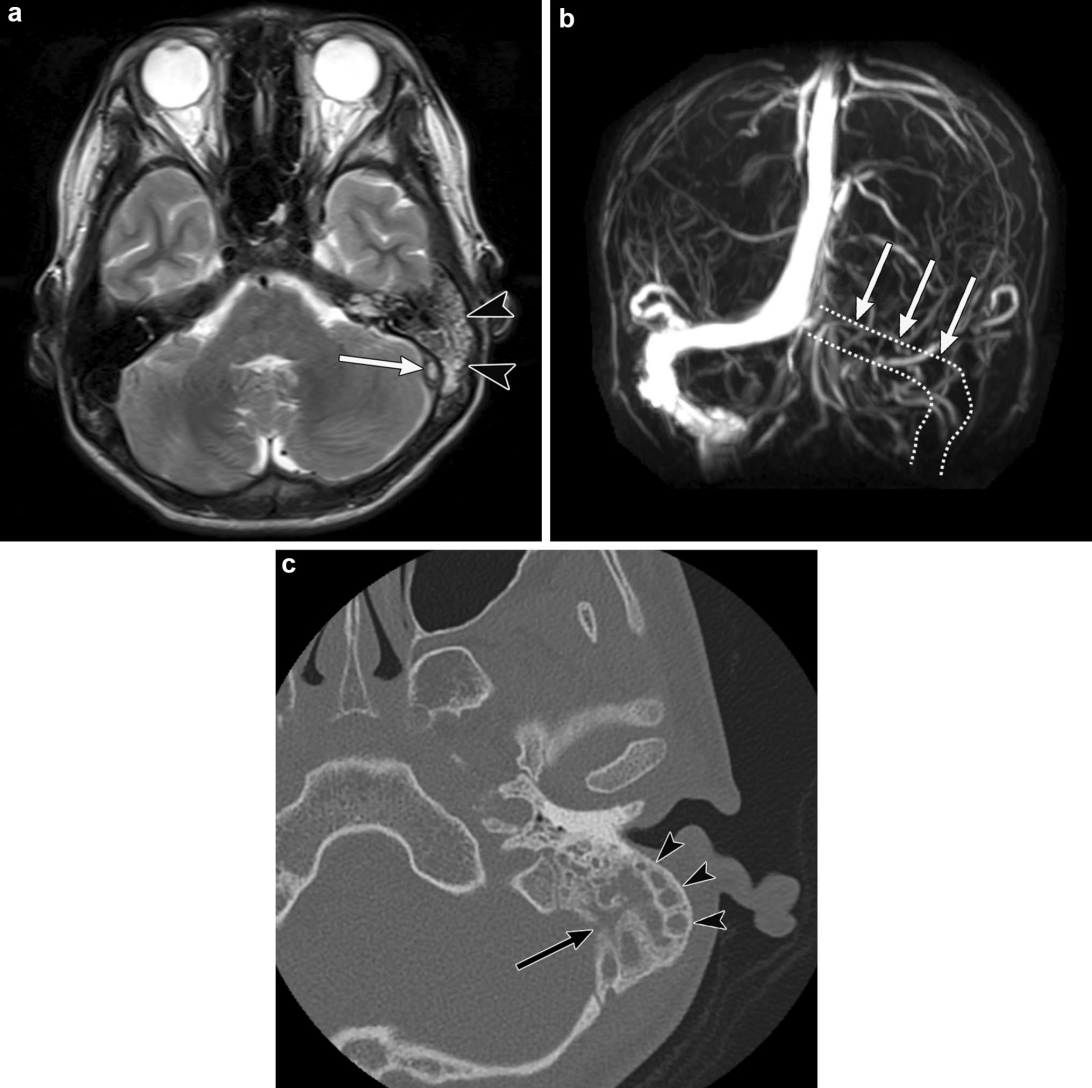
Mastoiditis in a 13-year-old boy with bacterial meningitis. a Axial T2-weighted magnetic resonance (MR) image shows fluid collection in the left mastoid bone (arrowheads). The left sigmoid sinus shows the absence of a flow void, suggestive of sinus thrombosis (white arrow). b Maximum-intensity projection of a contrast-enhanced MR venogram shows a complete thrombosis of the left sigmoid sinus to the left transverse sinus (white arrows). c Axial computed tomography (bone window) shows fluid accumulation in the left mastoid air cells (arrowheads). The medial mastoid wall shows lytic changes (black arrow)
